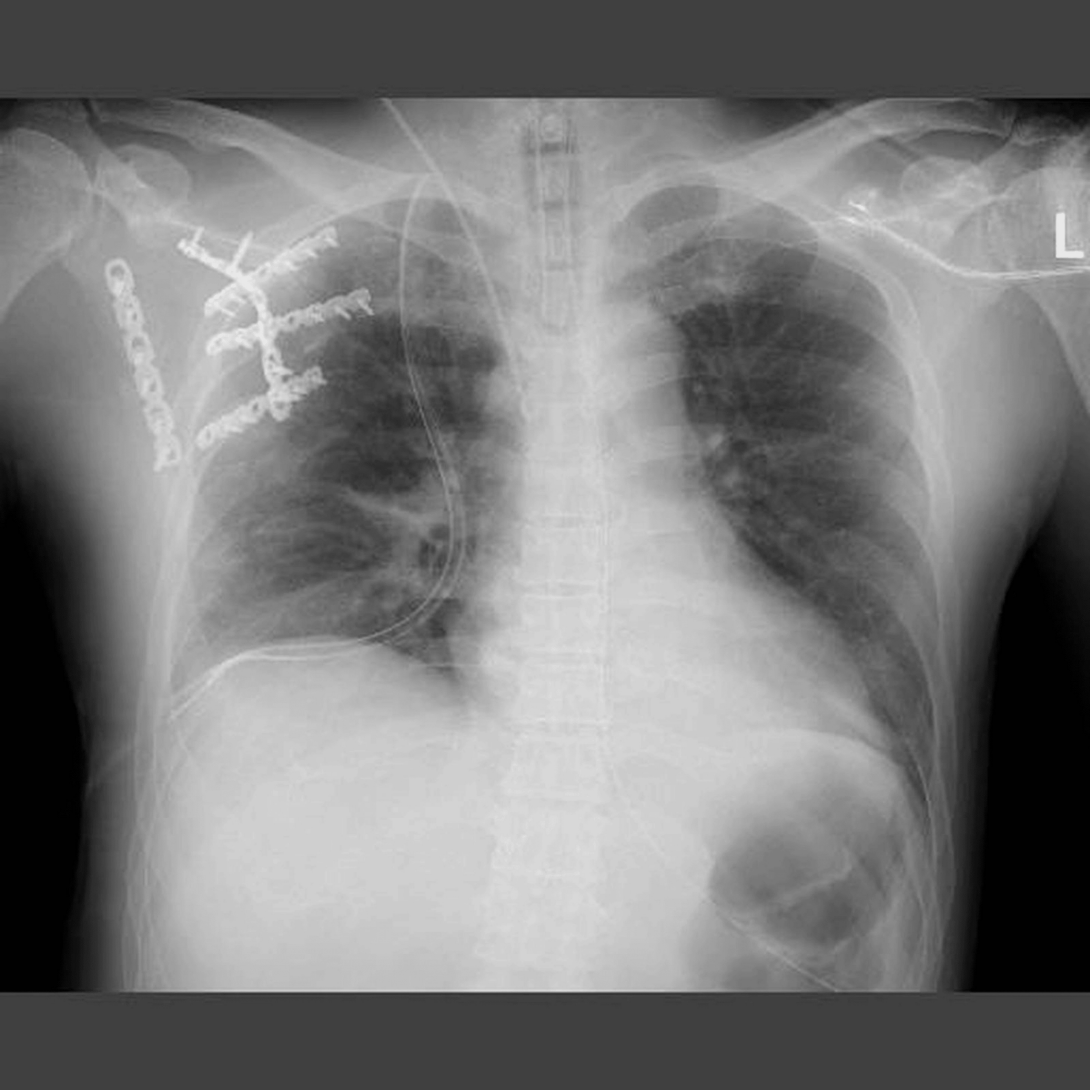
Postoperative chest and scapula X-ray images
